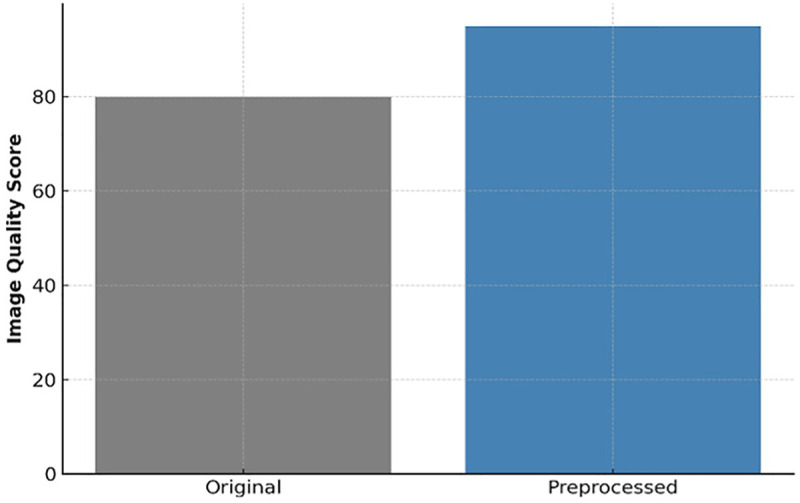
Sample panoramic radiographs original & preprocessed (256 × 256, normalized).
